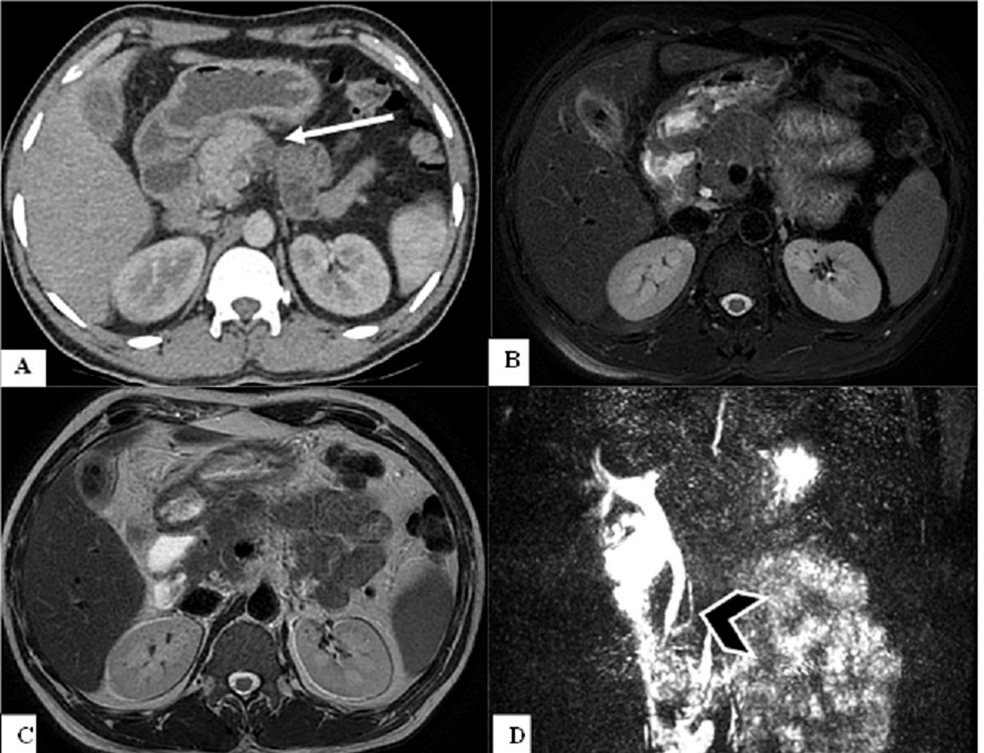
Acute pancreatitis in a case of partial agenesis of the dorsal pancreas. Contrast-enhanced computed tomography of the abdomen (A) showing heterogeneity in the visualized part of the head (white arrow). Axial T2 fat-suppressed image (B) and axial T2-weighted imaging (C) showing non-visualization of part of the body and tail of the pancreas with mild surrounding fat stranding. Magnetic resonance cholangiopancreatography (D) showing the short main pancreatic duct (chevron arrow). Serum amylase and lipase levels of the patient were raised.
